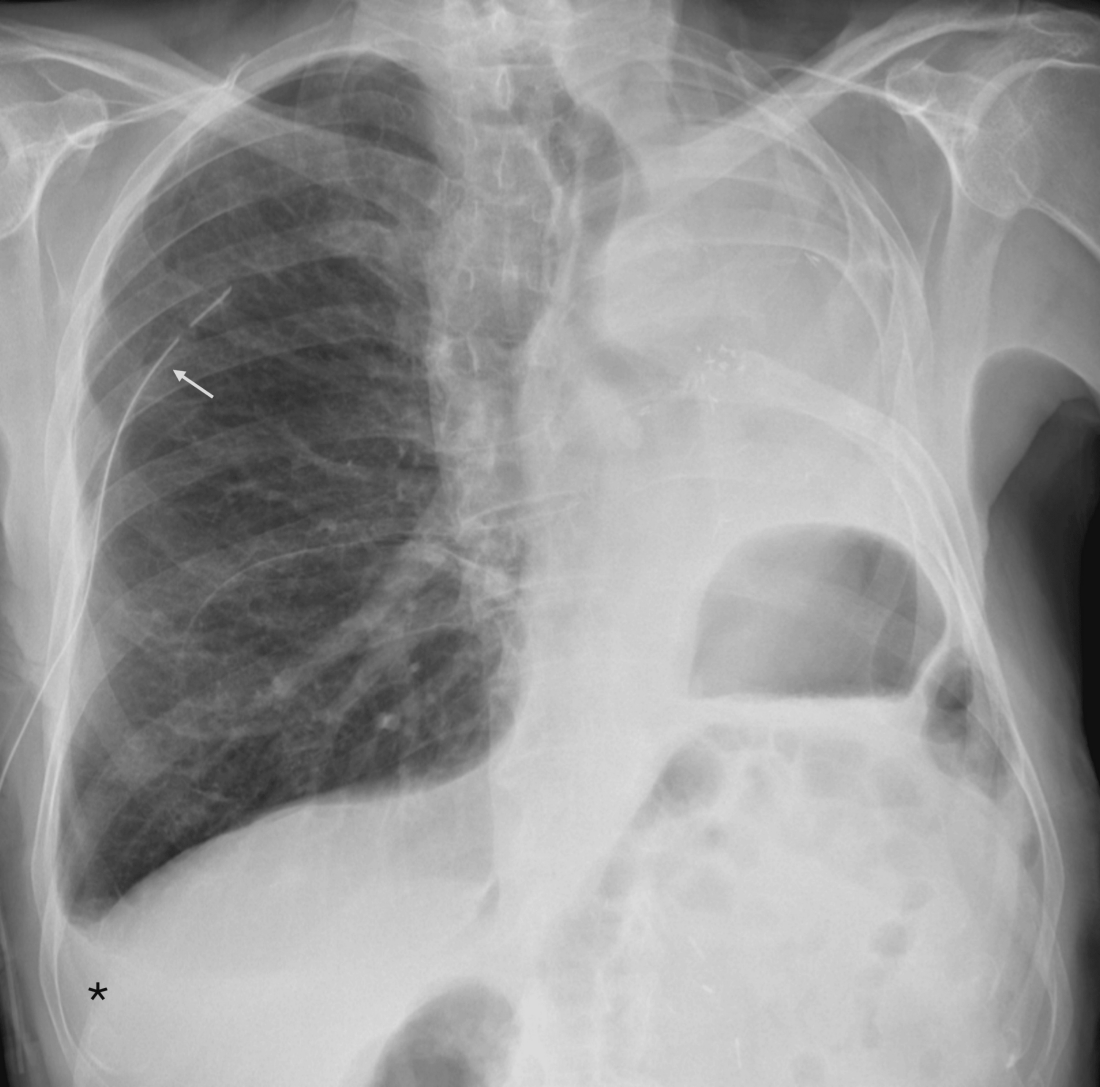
Post-pleurodesis chest radiography. Chest radiograph after talc pleurodesis showing full lung expansion. The asterisk indicates a small residual pleural effusion. The arrow denotes the chest tube in place.
